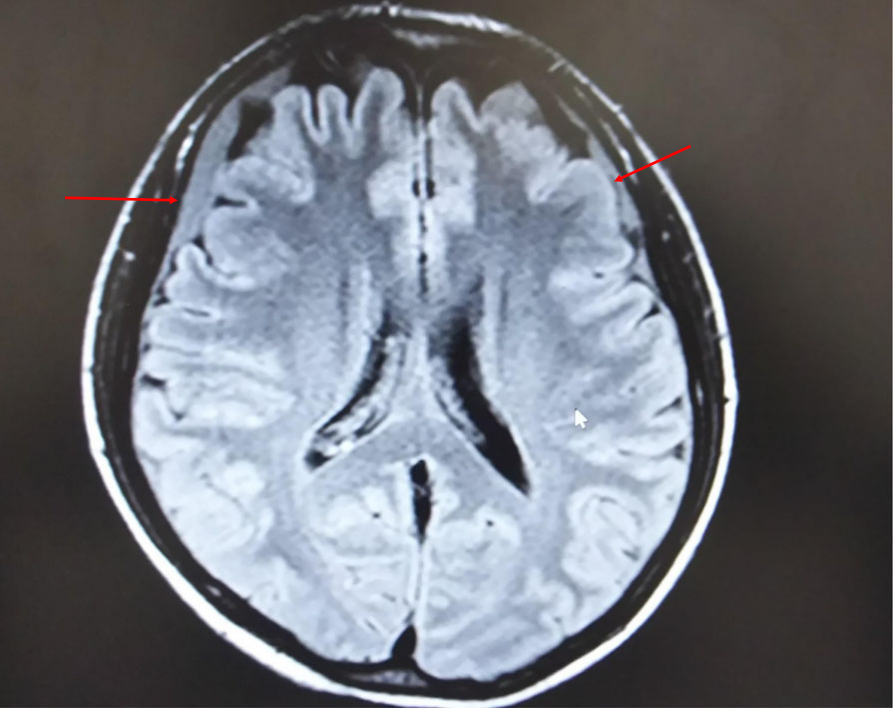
Magnetic resonance imaging performed 4 days after cesarean delivery. A bilateral subdural hematoma is shown (red arrow)
